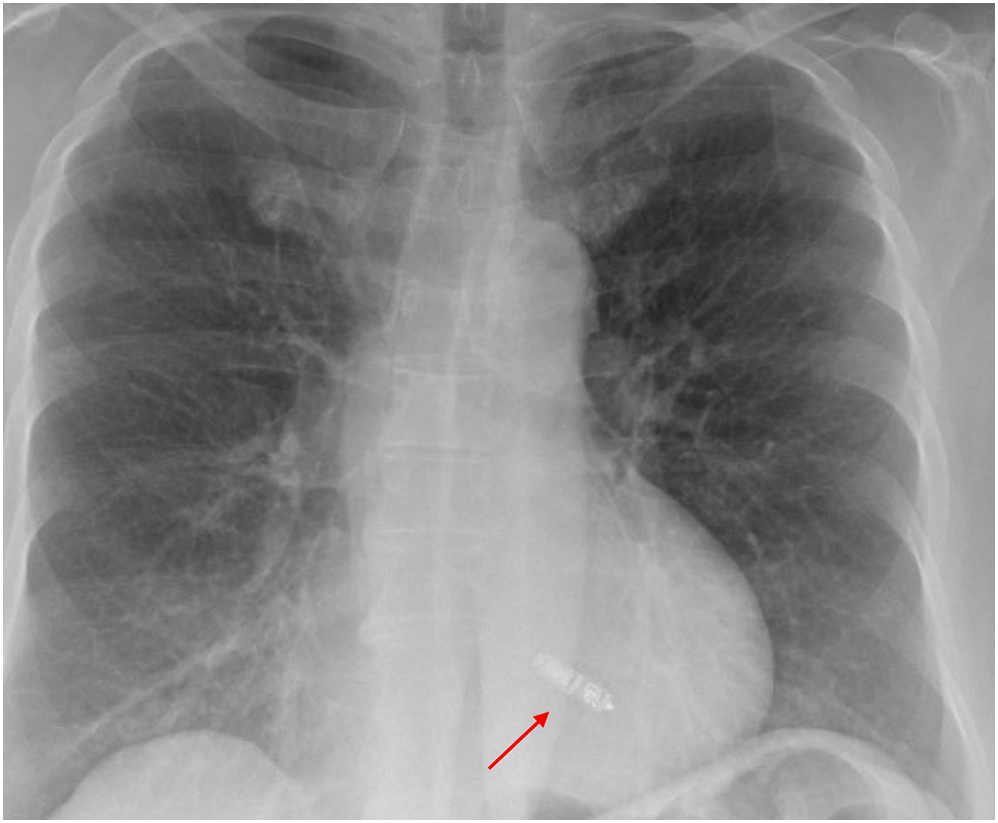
Chest x-ray demonstrating a Medtronic Micra leadless pacemaker implant (red arrow).
